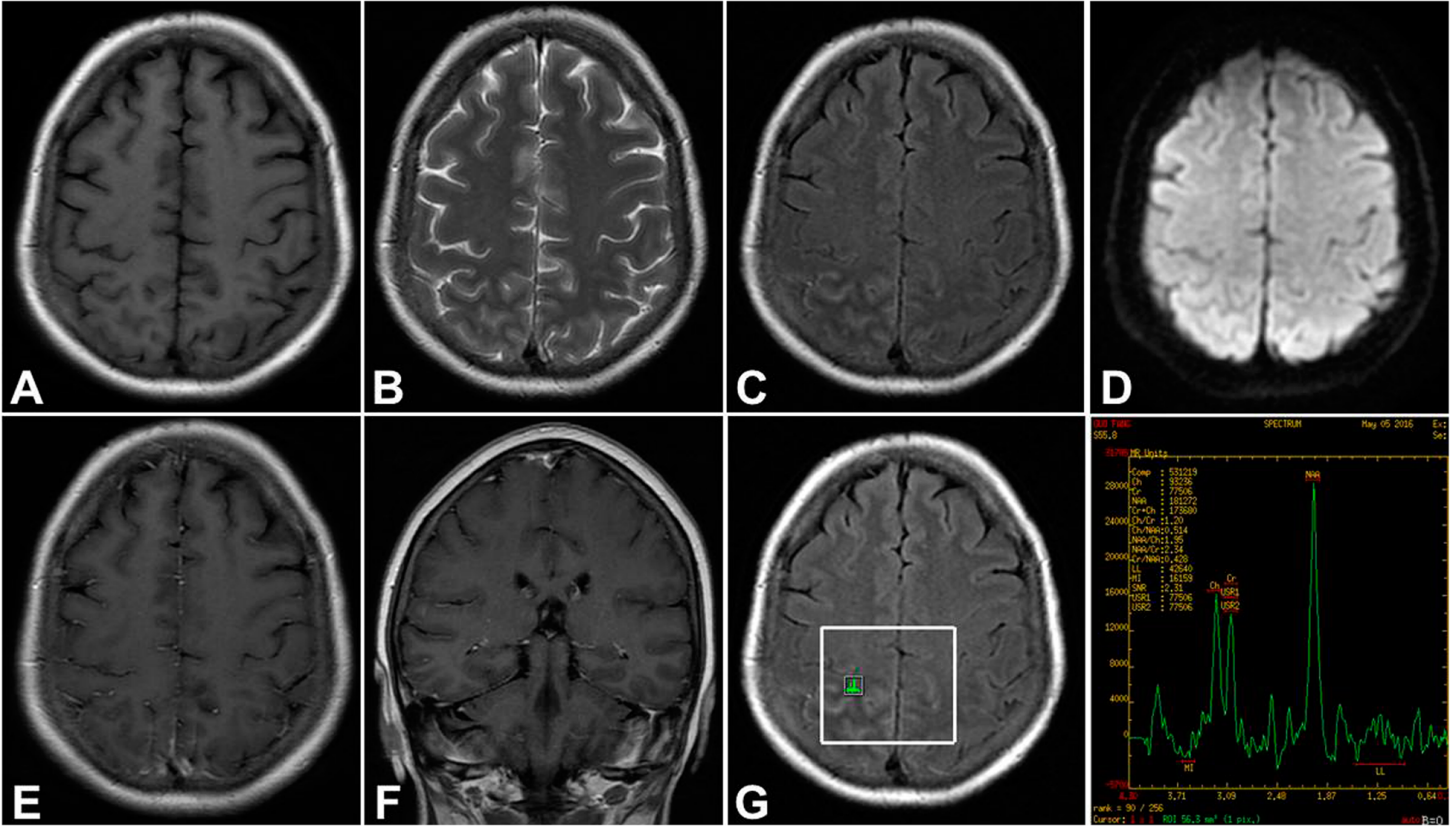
After treatment for 6 weeks, follow-up MRI showed nearly complete regression of lesion on *right* parietal lobe on T1-weighted (a), T2-weighted images (b), FLAIR (c), and still normal signal on DWI (d). MRI enhancement showed that the previous slim contrast enhancement almostly disappeared (e) and (f). MRS also showed normal NAA, Cr and Cho peak of previous lesion (g)
